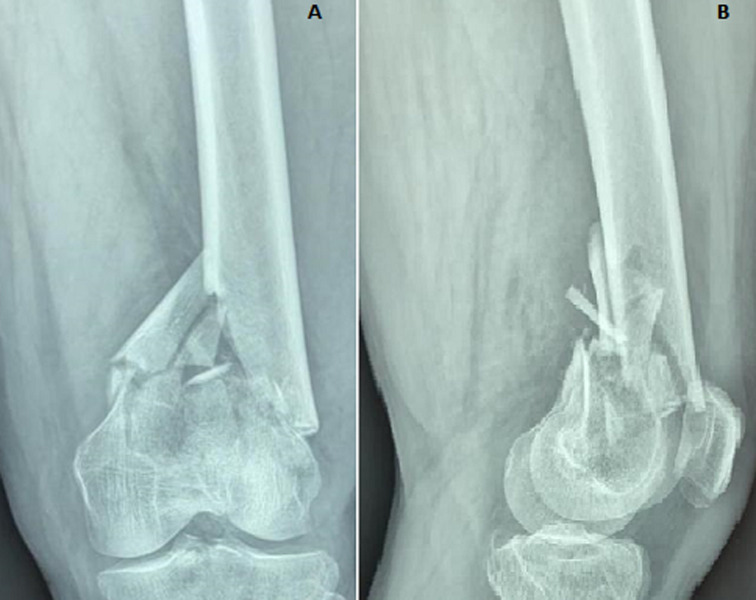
anteroposterior (A) and lateral (B) radiographs of a C2 type distal femoral fracture
